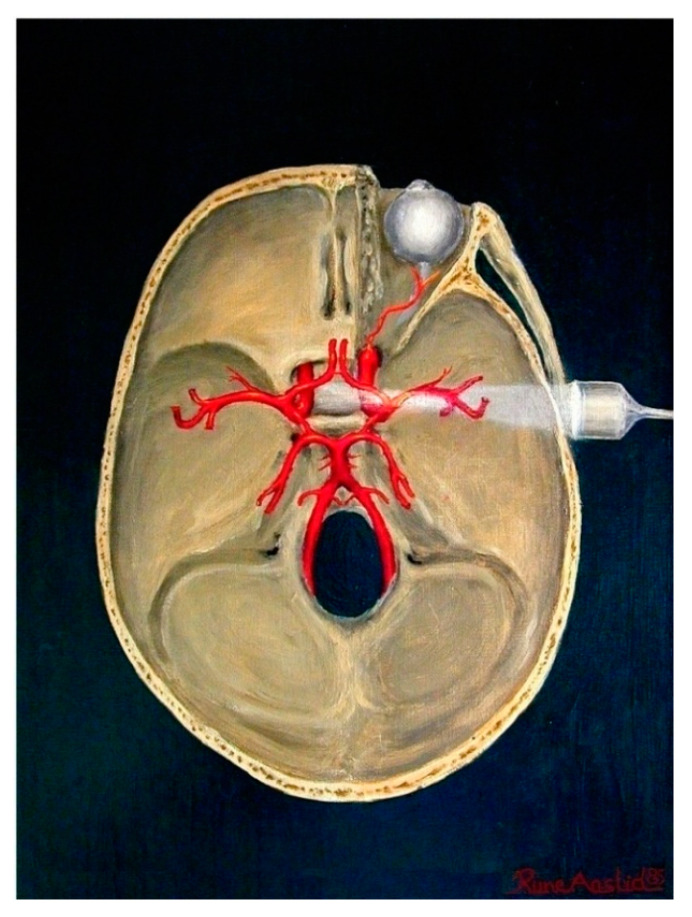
Principle of transcranial MCA Doppler ultrasound [22].
